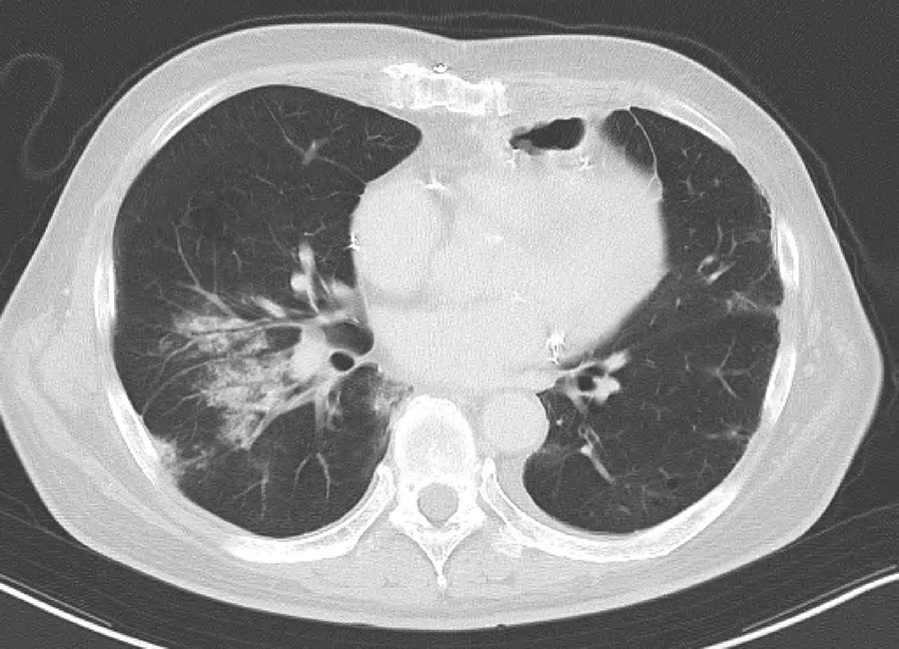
CT showing localized ground-glass opacities in both lungs at admission in Case 2. CT, computed tomography
